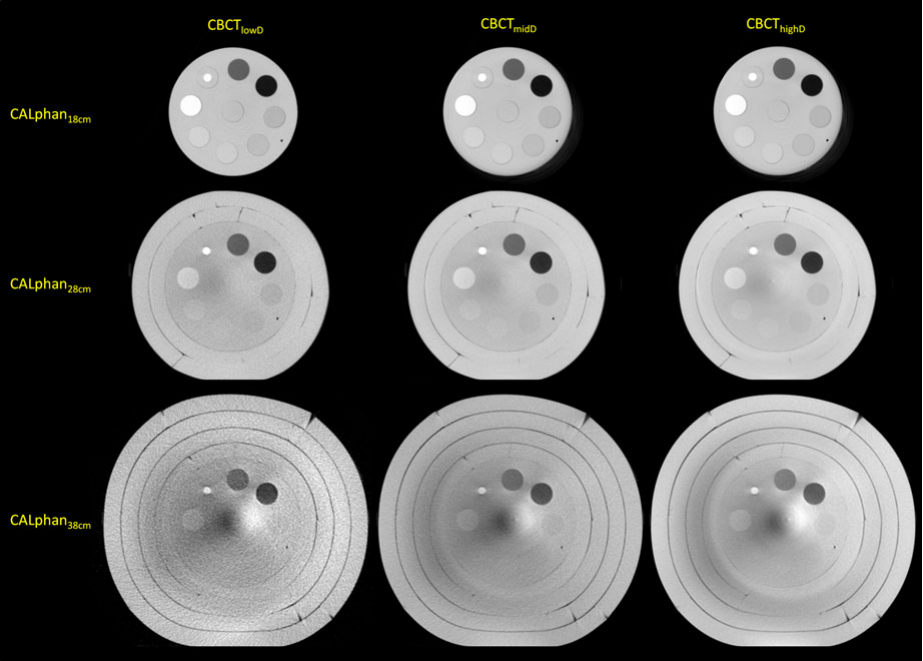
Axial images acquired with CBCT_lowD_, CBCT_midD_, and CBCT_highD_ (left to right) for CALphan_18 cm_, CALphan_28 cm_, and CALphan_38 cm_ (top to bottom).
